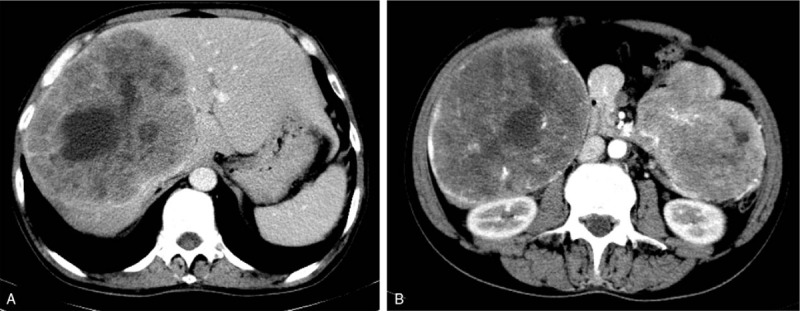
Tumor size (longest diameter × shortest diameter) in computed tomography (preoperative). (A) 12.4 × 14.5 cm. (B) Liver 12.8 × 10.7 cm. Intestinal tumor 10.5 × 7 cm.
